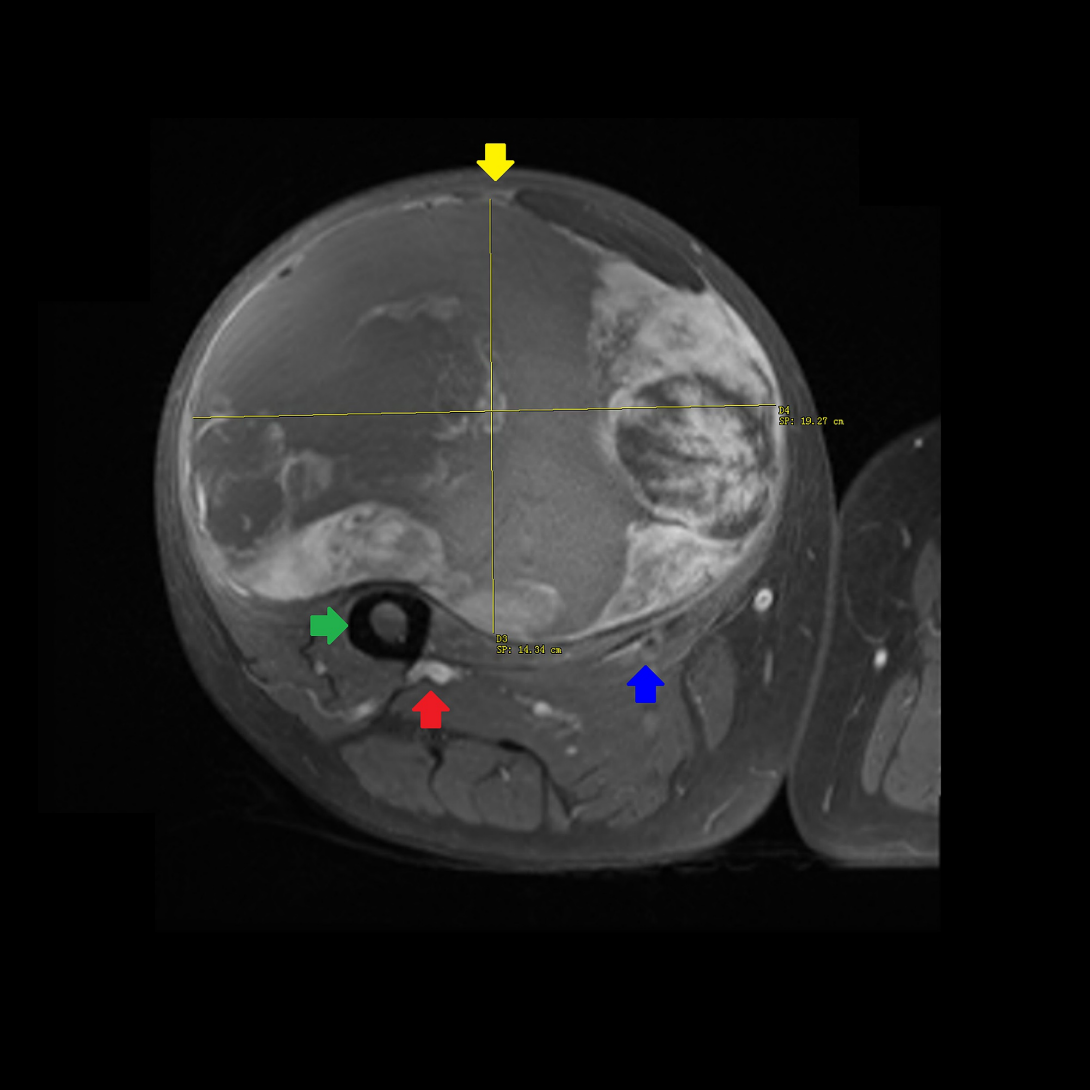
T1-weighted turbo spin echo MRI with gadolinium contrast of the right thigh in axial plane. An anterior compartment mass (yellow arrow) measuring 26 × 19 × 14 cm. The central non-lipogenic component measures 24 × 19 × 14 cm and demonstrates irregular, inhomogeneous nodular peripheral enhancement. Peripheral fat densities are present. There is no invasion of the femur (green arrow), profunda femoris artery/vein (red arrow), or superficial femoral artery/vein and posterior division of the femoral nerve (blue arrow). The rectus femoris muscle is completely encased by the tumor. The sartorius, vastus medialis, and vastus intermedius muscles are almost completely encased by the tumor. The vastus lateralis is partially encased and posteriorly displaced by the tumor. There is no tumor involvement beyond the anterior thigh compartment.
